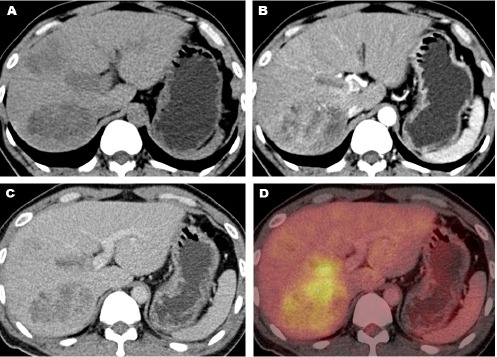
The contrast-enhanced FDG PET/CT shows a liver tumor with heterogeneous contrast enhancement and increased FDG uptake, adjacent to the right expansile thrombosed portal vein. The thrombus appeared hypodense in the non-enhanced CT (A). It showed perithrombus contrast enhancement in the arterial phase CT (B), appeared washout in the portal venous phase CT (C), and showed an increased FDG uptake in the PET/CT image (D)
